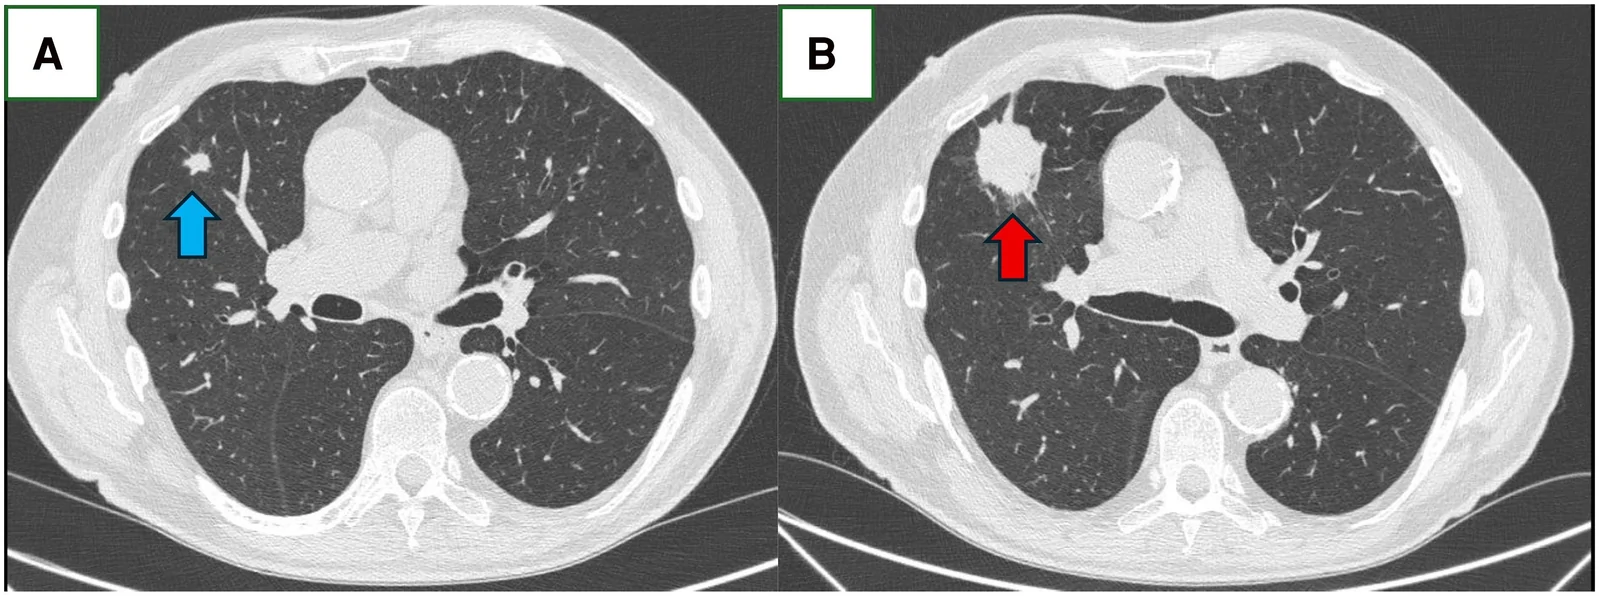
High-resolution CT of right pulmonary nodule. (A) High-resolution chest CT showing an irregular pulmonary nodule measuring 12 × 15 × 17 mm in the right lung (arrow). (B) High-resolution chest CT performed after 3 months showing enlargement of the pulmonary nodule to 44 × 32 × 35 mm (arrow).
